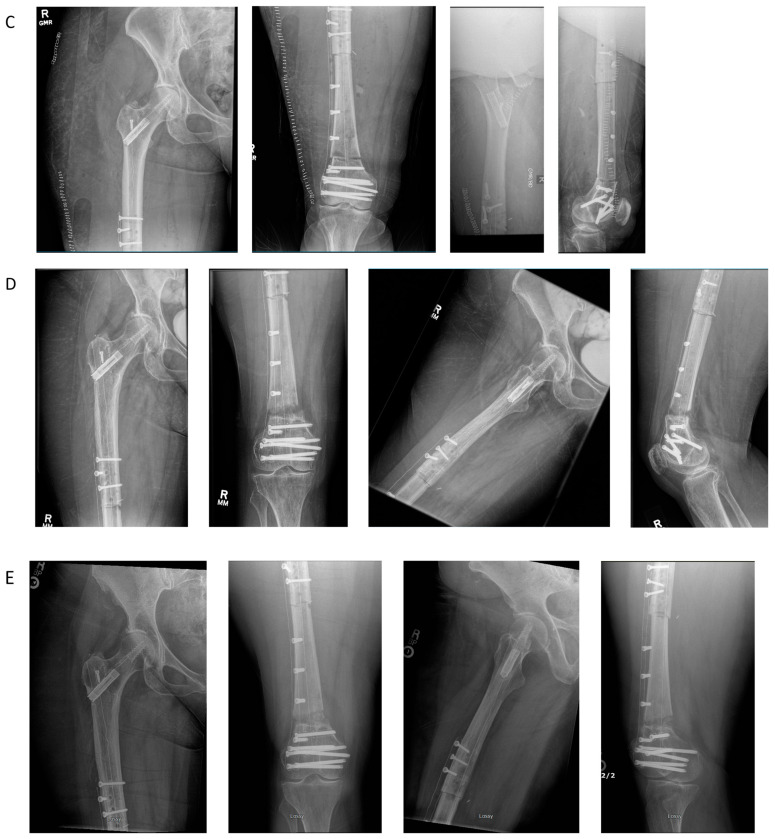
(A) Pre-operative anteroposterior (AP) and lateral radiographs of the right distal femur, demonstrating a lesion in the distal metadiaphysis. (B) Pre-operative post-contrast, T1 fat-suppressed coronal, sagittal, and axial magnetic resonance (MR) images of the distal femur further characterizing the high-grade dedifferentiated chondrosarcoma. Note is made of the cortical breach and extramedullary soft-tissue component. (C) Immediate post-operative AP and lateral radiographs of the right femur, demstatus post intercalary resection with allograft reconstruction utilizing a carbon fiber cephalomedullary nail and carbon fiber plate. (D) 15-month post-operative AP and lateral radiographs of the right femur, demonstrating allograft non-union and hardware loosening. (E) The 6-week post-operative AP and lateral radiographs of the right femur status-post removal of the carbon fiber plate and non-union revision open reduction internal fixation with iliac crest bone graft with use of a carbon fiber plate. The carbon fiber hardware facilitated clearer visualization of the allograft–native bone interface to assess for subsequent bony union.
